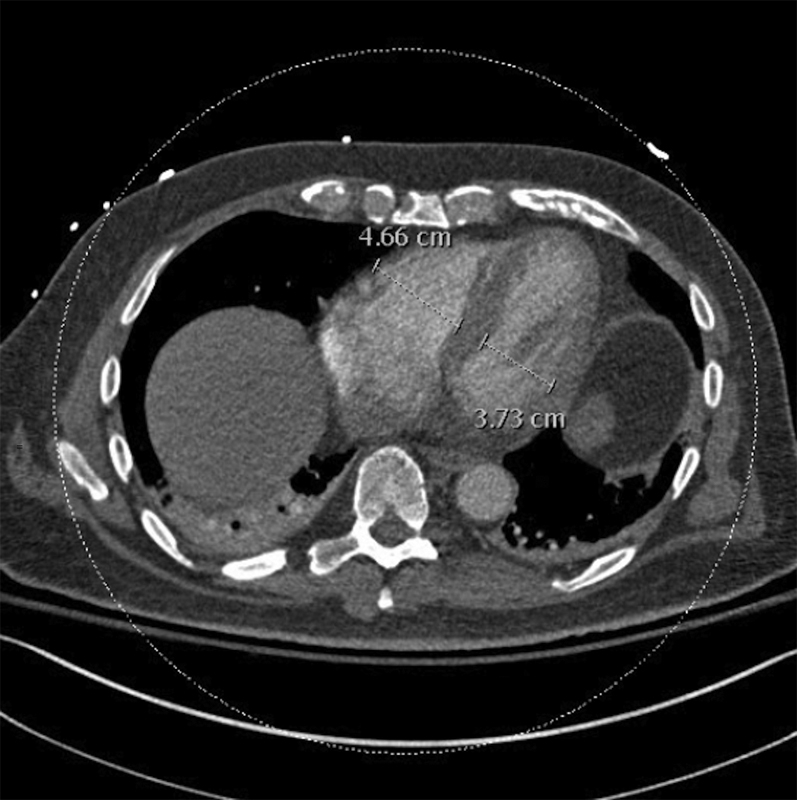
Computed Tomography Pulmonary Angiography Findings of Right Heart Strain Abnormal right ventricle: left ventricle ratio of 1.2.
